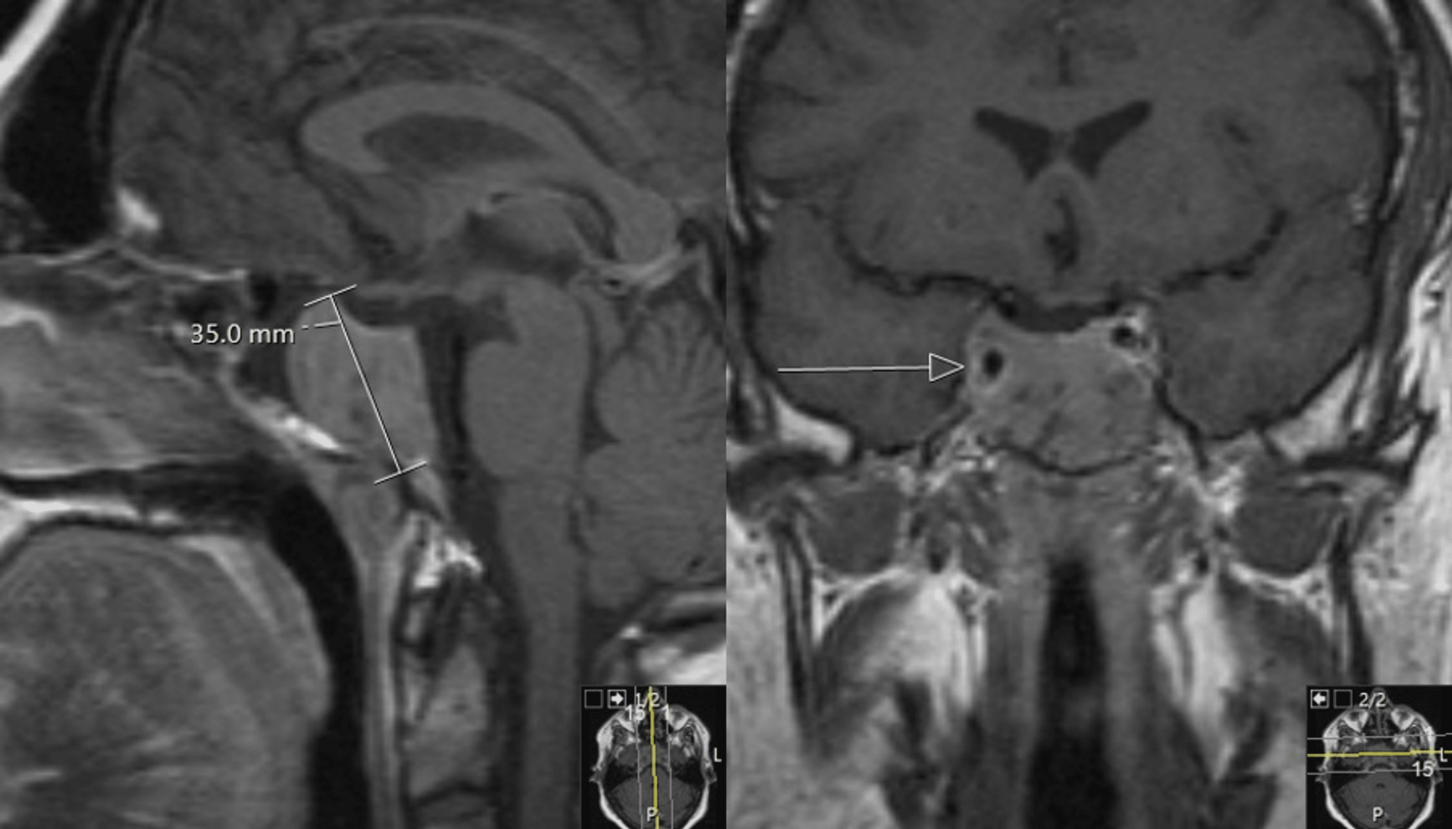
Macroprolactinoma Post-contrast T1 sagittal and coronal images (1.5 Tesla MRI scanner, Siemens Healthcare Laboratory Diagnostics). Giant invasive pituitary lesion replacing the sphenoid sinus and invading the right cavernous sinus (white arrow). Maximum diameter is 35 mm.
